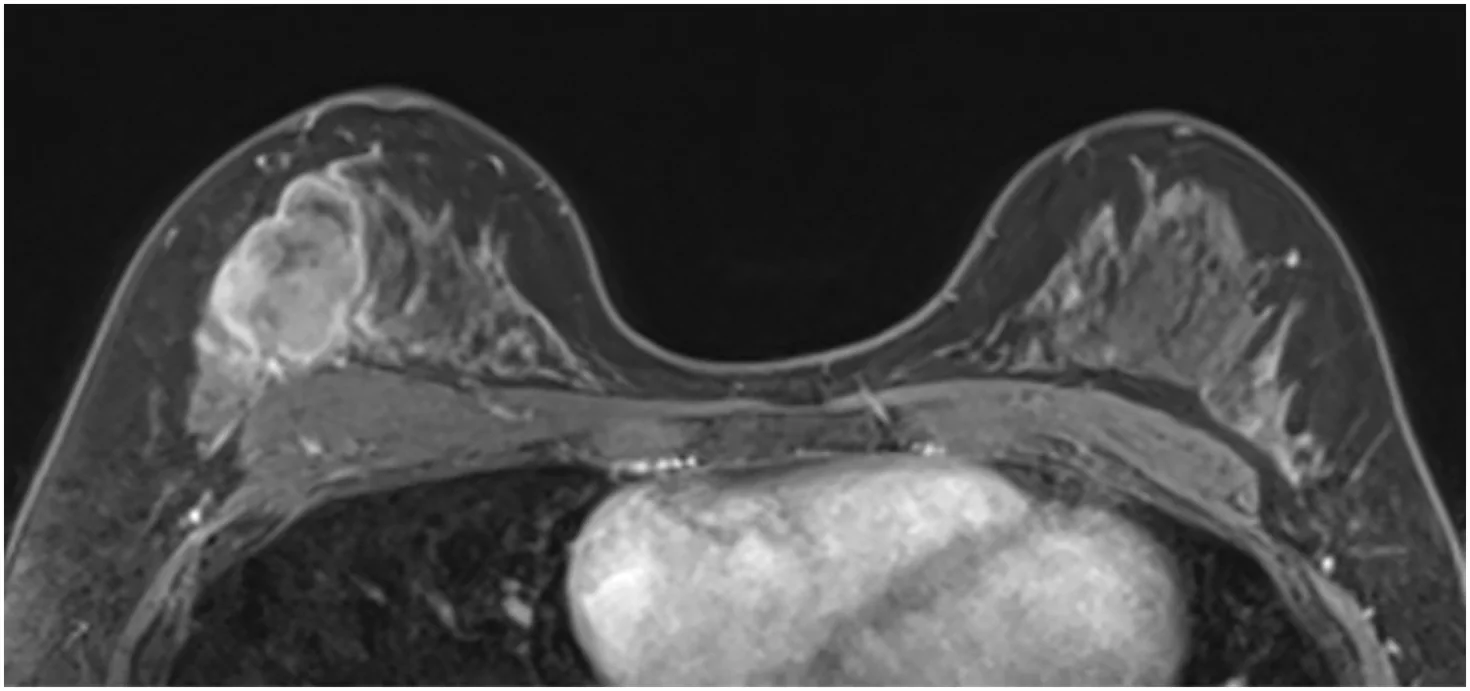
Contrast-enhanced MRI findings (before initiation of neoadjuvant chemotherapy). A solid lobulated mass measuring 38 mm with ring enhancement was observed in the right breast.
